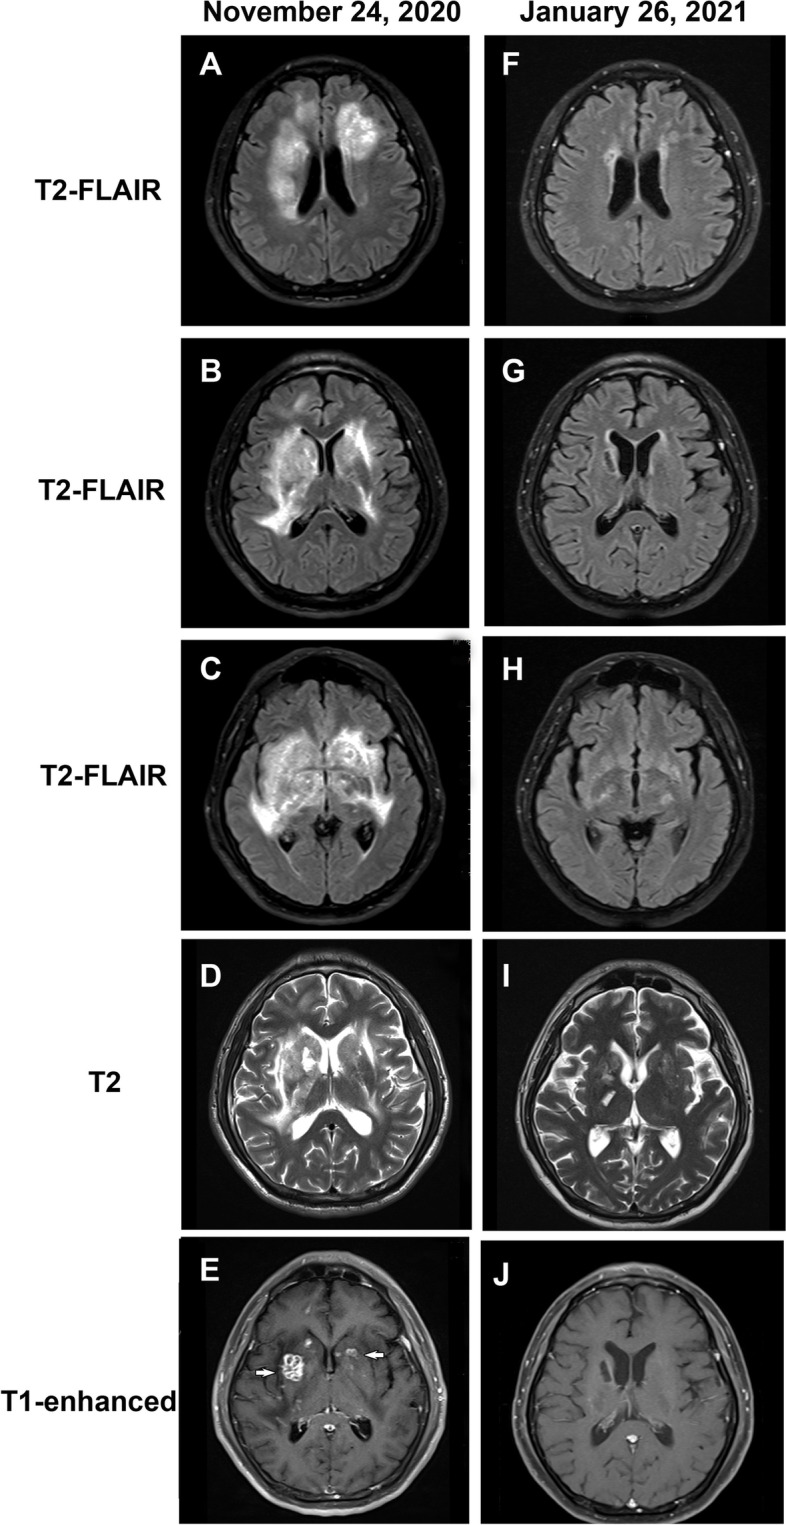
Neuroimaging features of the patient. A-E Brain MRI performed before the administration of corticosteroids. A-C T2-FLAIR images demonstrate multiple hyperintense lesions at different levels, which mainly located in the basal ganglia, internal capsule, external capsule, periventricular, corona radiata, frontal and temporal lobes. D Conventional T2-weighted image shows multiple lesions with poorly defined boundaries in the bilateral basal ganglia. E Gadolinium-enhanced T1-weighted image shows multiple ring-shaped zones of peripheral contrast enhancement in the right basal ganglia and nodular contrast enhancement in the bilateral basal ganglia (white arrows). F-J Brain MRI performed on the day 54 after treatment with corticosteroids. F-H T2-FLAIR images show almost complete disappearance of previous lesions (I) Conventional T2-weighted image shows that lesions in the bilateral basal ganglia were significantly reduced. J Gadolinium-enhanced T1-weighted image shows most of the contrast-enhancing lesions disappeared. Note: MRI = magnetic resonance imaging; FLAIR = fluid-attenuated inversion recovery
